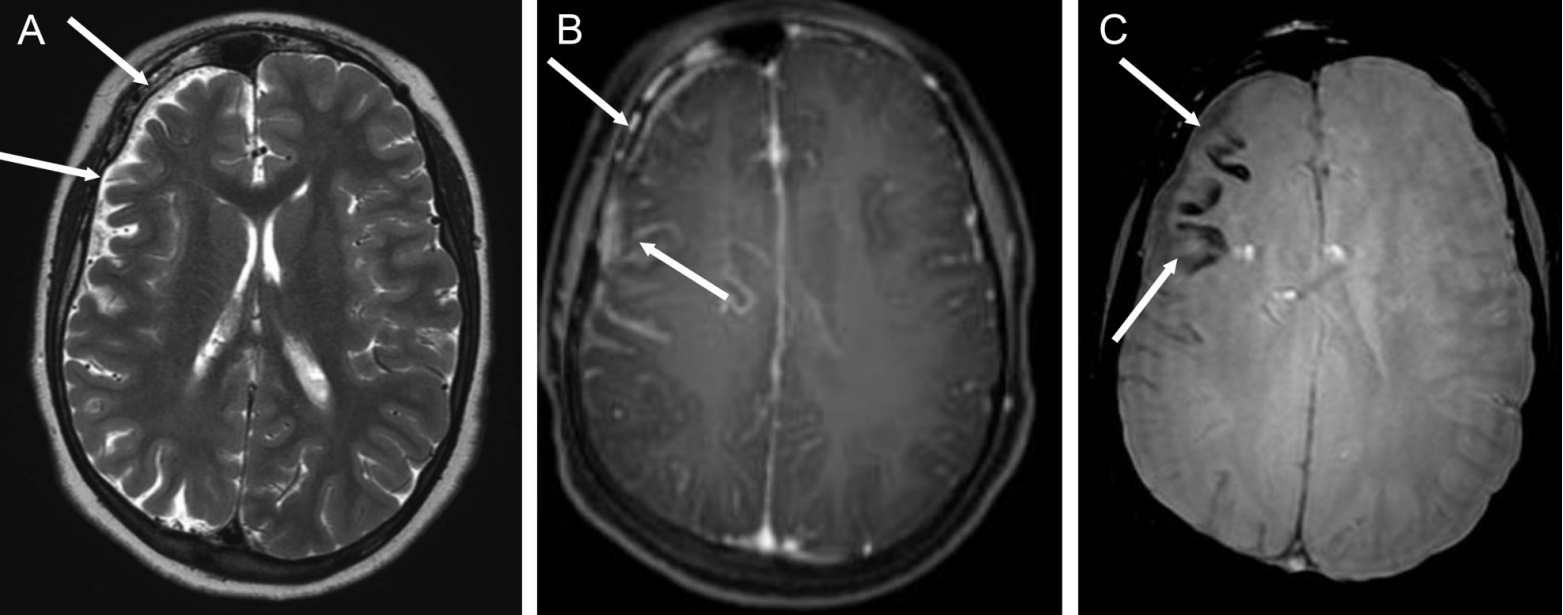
Sturge-Weber syndrome in a 14-year-old boy with multiple epileptic foci in the right cerebral hemisphere (video-EEG) and chronic partial epilepsy. A) An axial T2-weighted image showing right cerebral hemiatrophy (white arrows). B) An axial T1-weighted sequence after gadolinium injection, demonstrating leptomeningeal enhancement of the fronto-parietal region (white arrows). C) Axial SWI revealing cortical calcification in the frontal lobe, appearing as signal voids (white arrows).
